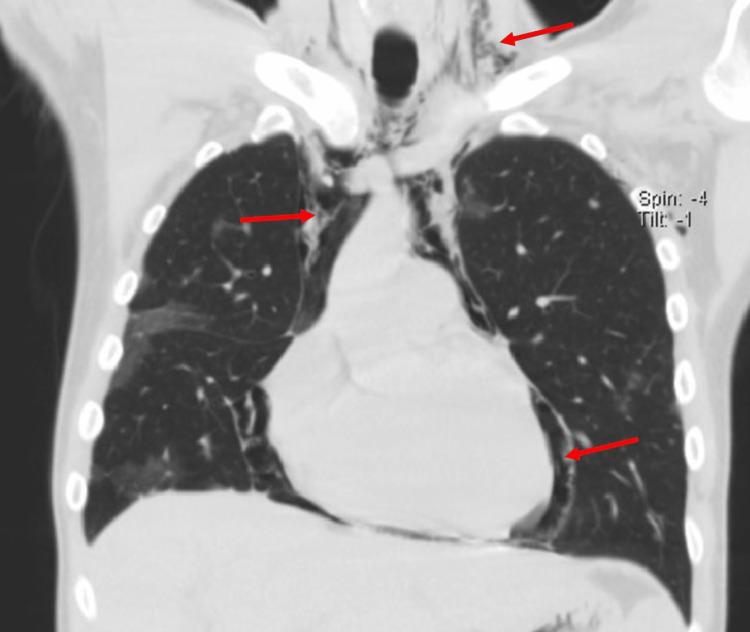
CT pulmonary angiogram (coronal view): red arrows indicate the presence of air leak syndrome.
